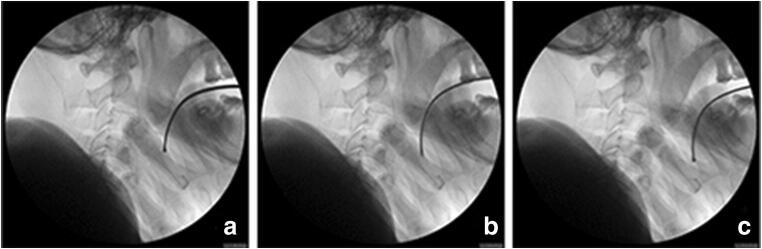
X-ray of patients with tongue root holder in this study. a The tongue root holder was inserted into the midline of the oral pharynx and placed on the dorsum of the tongue. b The jaw thrust maneuver was performed. c The handle was then lifted with an upwards and forward motion, pulling the tongue anteriorly and away from the soft palate and the posterior pharynx
